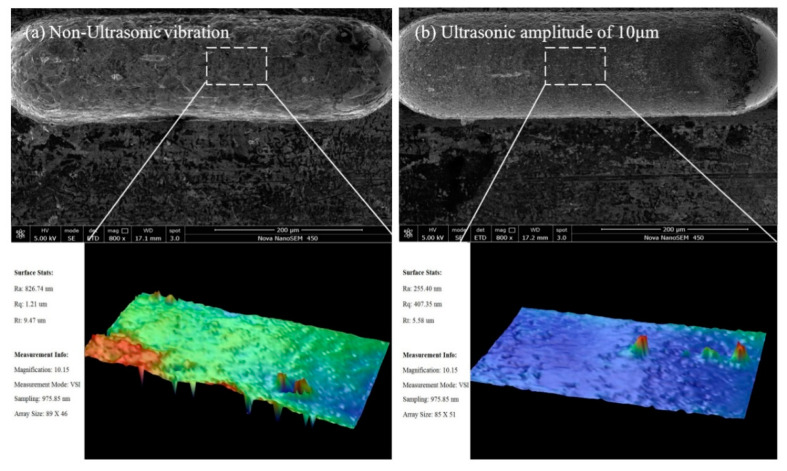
Comparison of surface roughness: (a) non-ultrasonic vibration; and (b) ultrasonic vibration of 10 µm.
